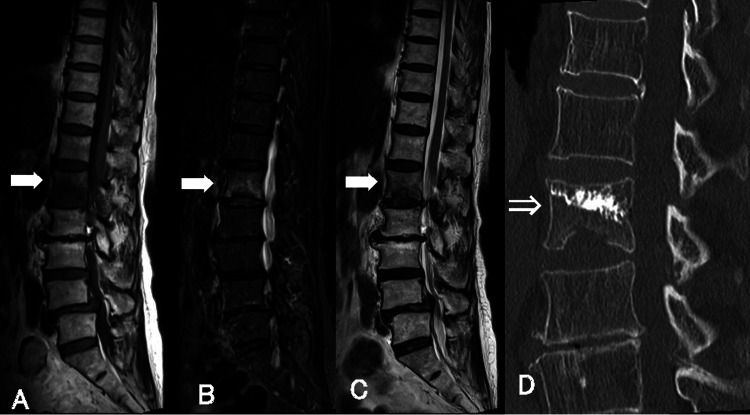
Preoperative MRI and CT after vertebroplasty. A-C: Thoracolumbar MRI showing a new vertebral fracture of the L1 vertebral body (white-painted arrow). A: T1WI; B: STIR; C: T2WI. D: Thoracolumbar CT showing augmentation of polymethyl methacrylate (white-open arrow). MRI: magnetic resonance imaging; CT: computed tomography; WI: weighted imaging; STIR: short-TI inversion recovery
